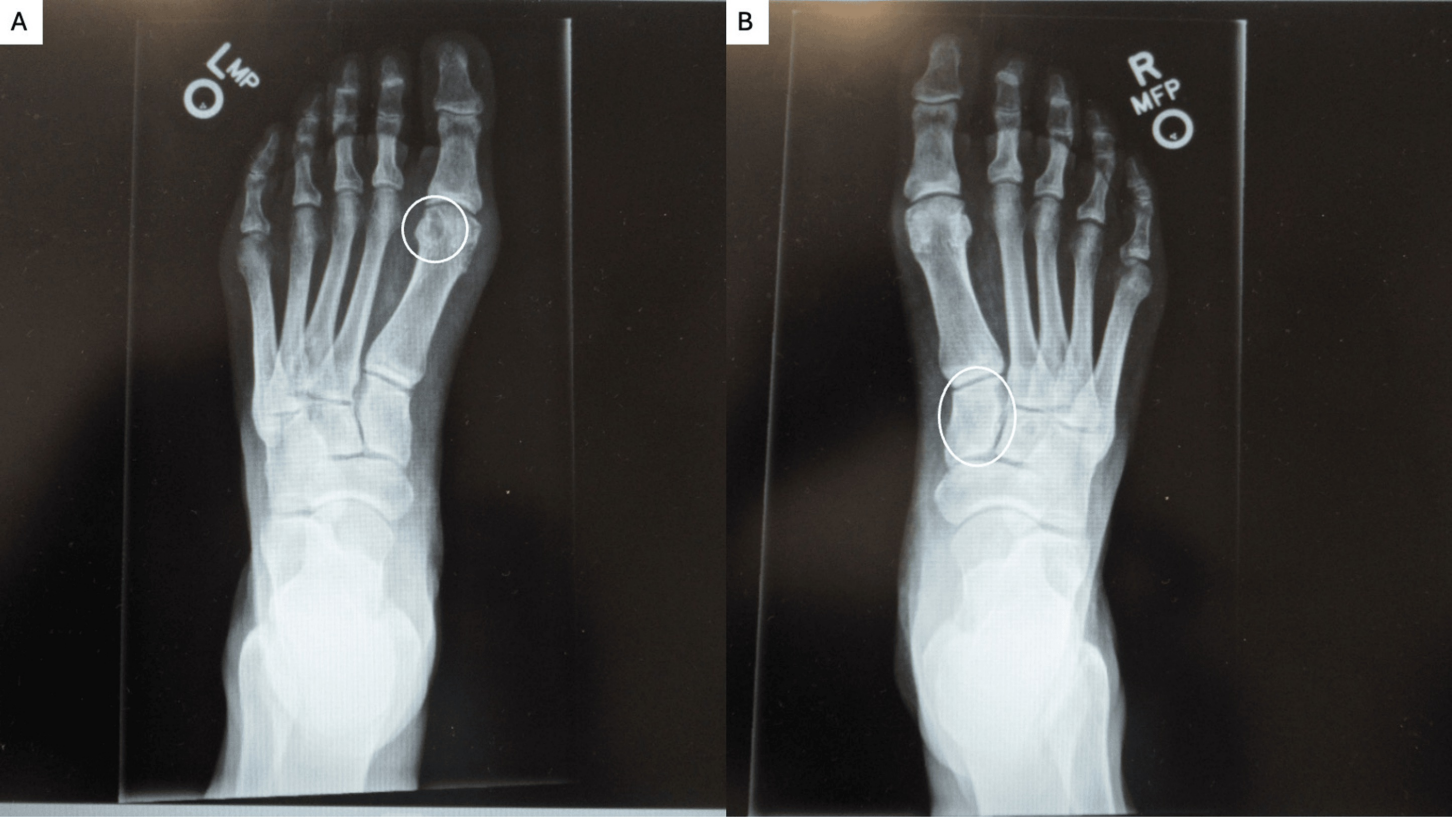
Radiograph of the patient's feet with gout. Roentgenograms showing the top view of the left (A) and right (B) foot. A well-defined erosion at the lateral aspect of the distal first metatarsal bone (within the hollow white oval) is noted on the left foot (A). A lucency, which was consistent with an erosion from a tophus, was present on the middle cuneiform (within the hollow white oval) of the right foot (B).
